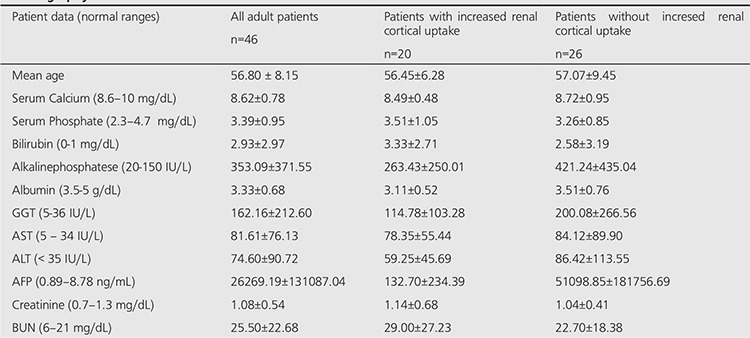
Biochemical finding in adult patients with and without increased renal cortical uptake at the time of bone scintigraphy
